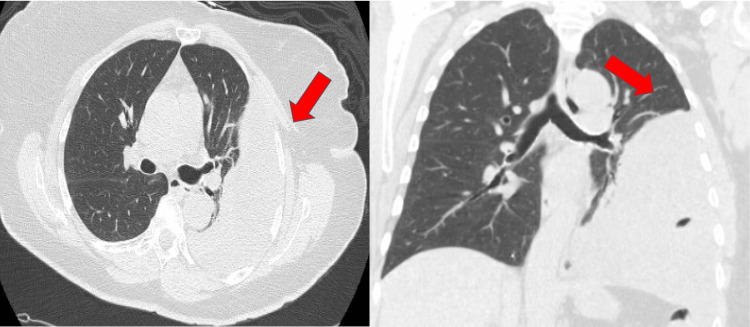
Computed tomography of the chest obtained for further evaluation of pleural disease showing complex pleural collection.
